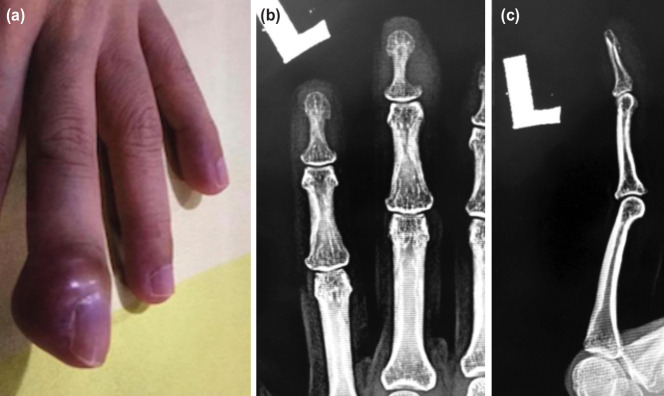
(a) Clinical picture of the left middle finger swelling and erythema mimicking an infection, (b and c) Lateral and AP radiograph of the left middle finger showing increase in soft tissue shadow and scalloping of the distal phalanx at two and half months after the onset of symptoms.
